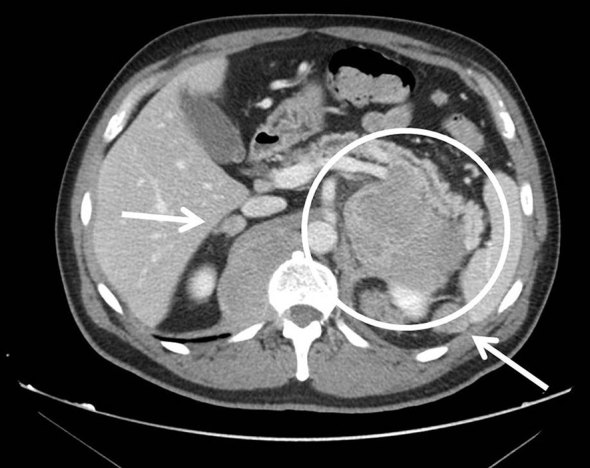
CT scan of the pelvis after i.v. contrast showing multiple intramuscular masses (circle).
